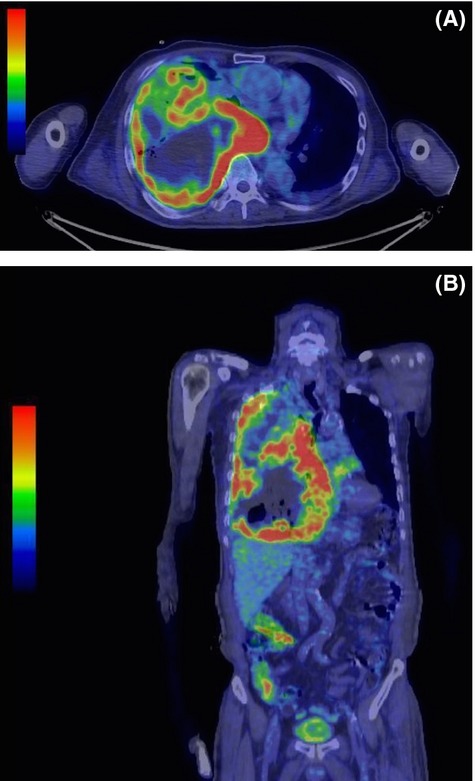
A positron emission tomography–computed tomography scan (A, B) revealed a high integration of fluorodeoxyglucose along the entire circumference of the pleura.
